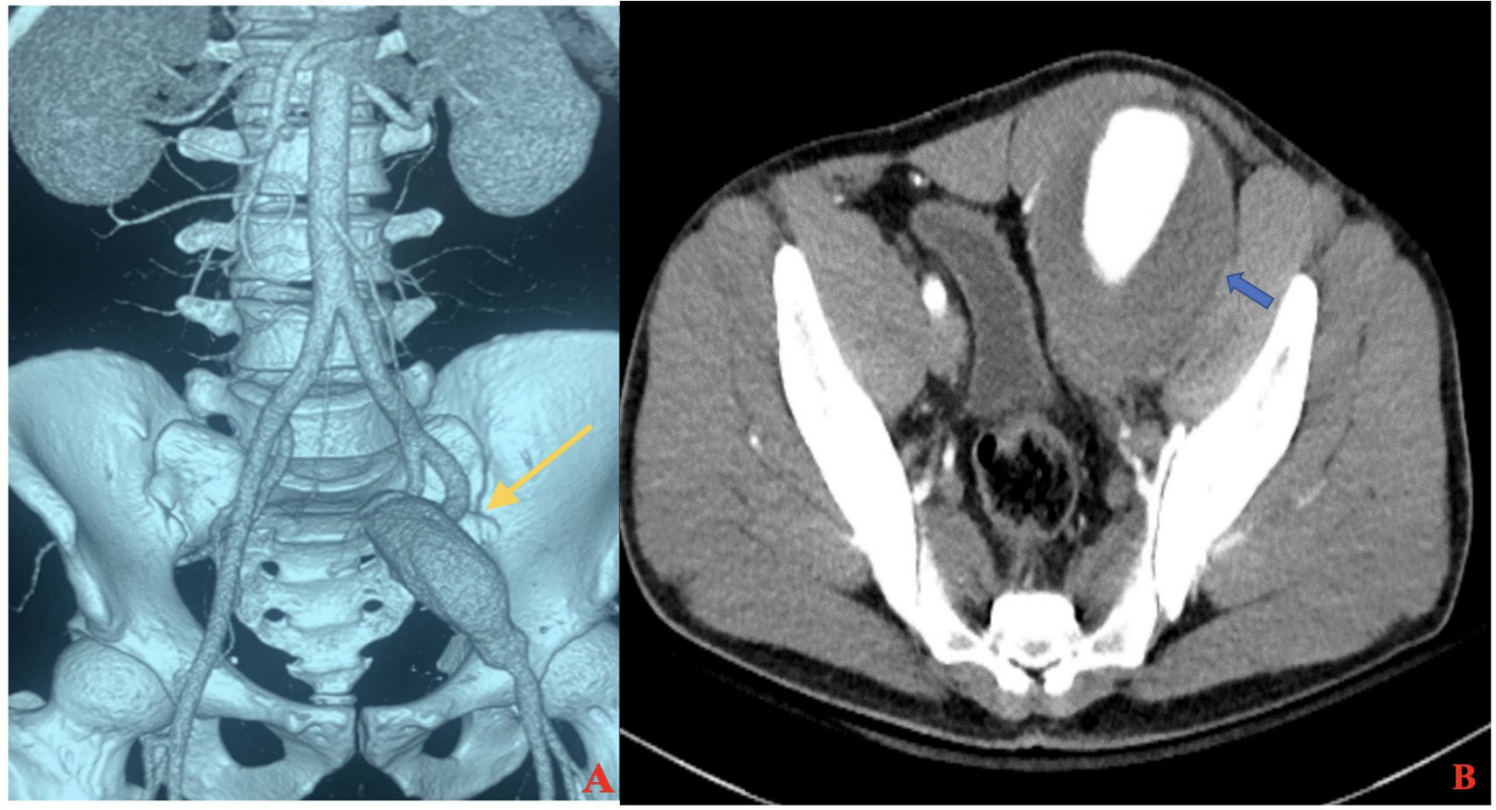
Computed tomography - (A) Volume rendered image- anteroposterior view showing left external iliac artery aneurysm (yellow arrow); (B) cross-sectional image showing aneurysmal dilation of left external iliac artery with intramural thrombus (blue arrow). The right external iliac artery and bilateral internal iliac arteries are normal.
